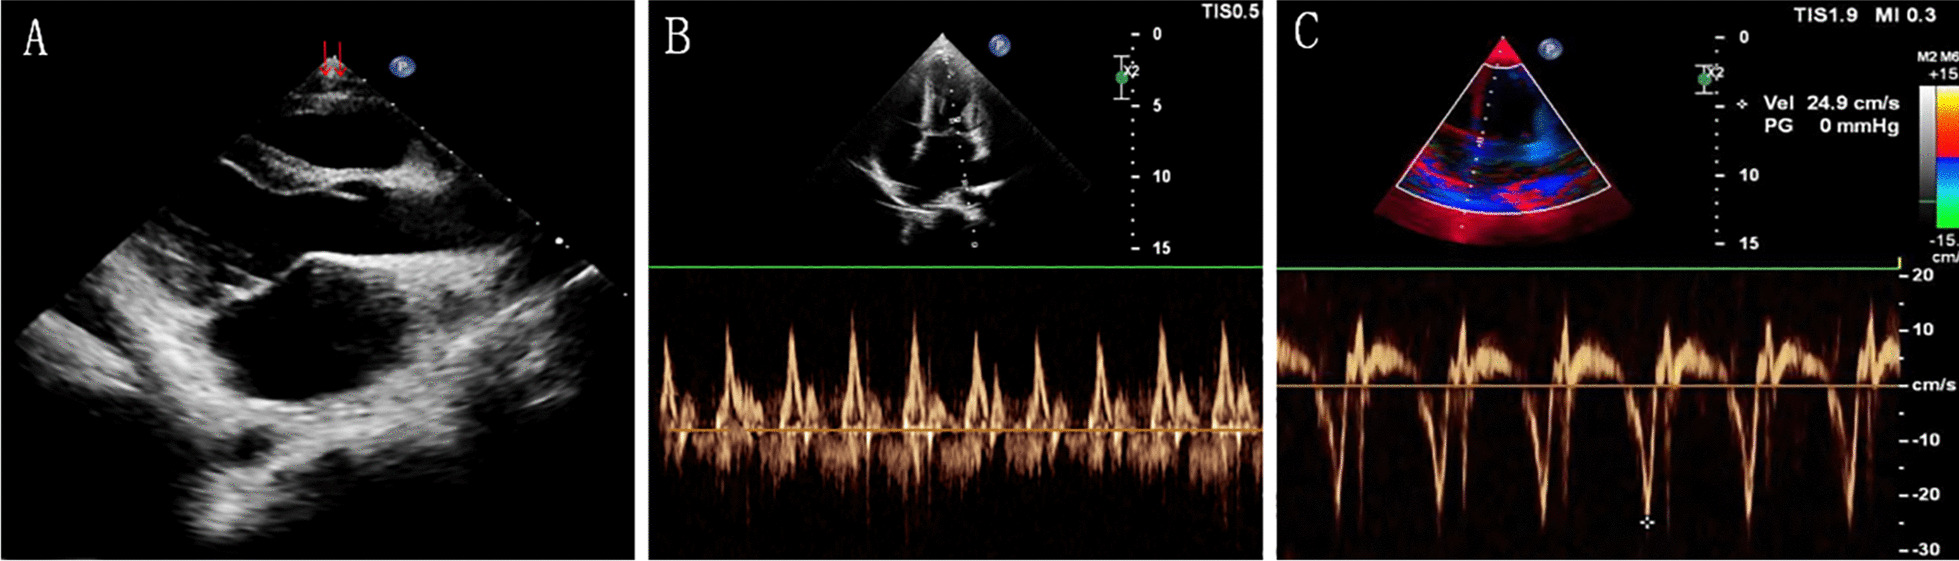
A Pericardial thickening in the long axis of the apex, anterior to the right ventricle (red arrow). B The flow velocity of the mitral valve increased with the variation of respiration. C Tissue Doppler showed no abnormal atrioventricular annulus velocity
